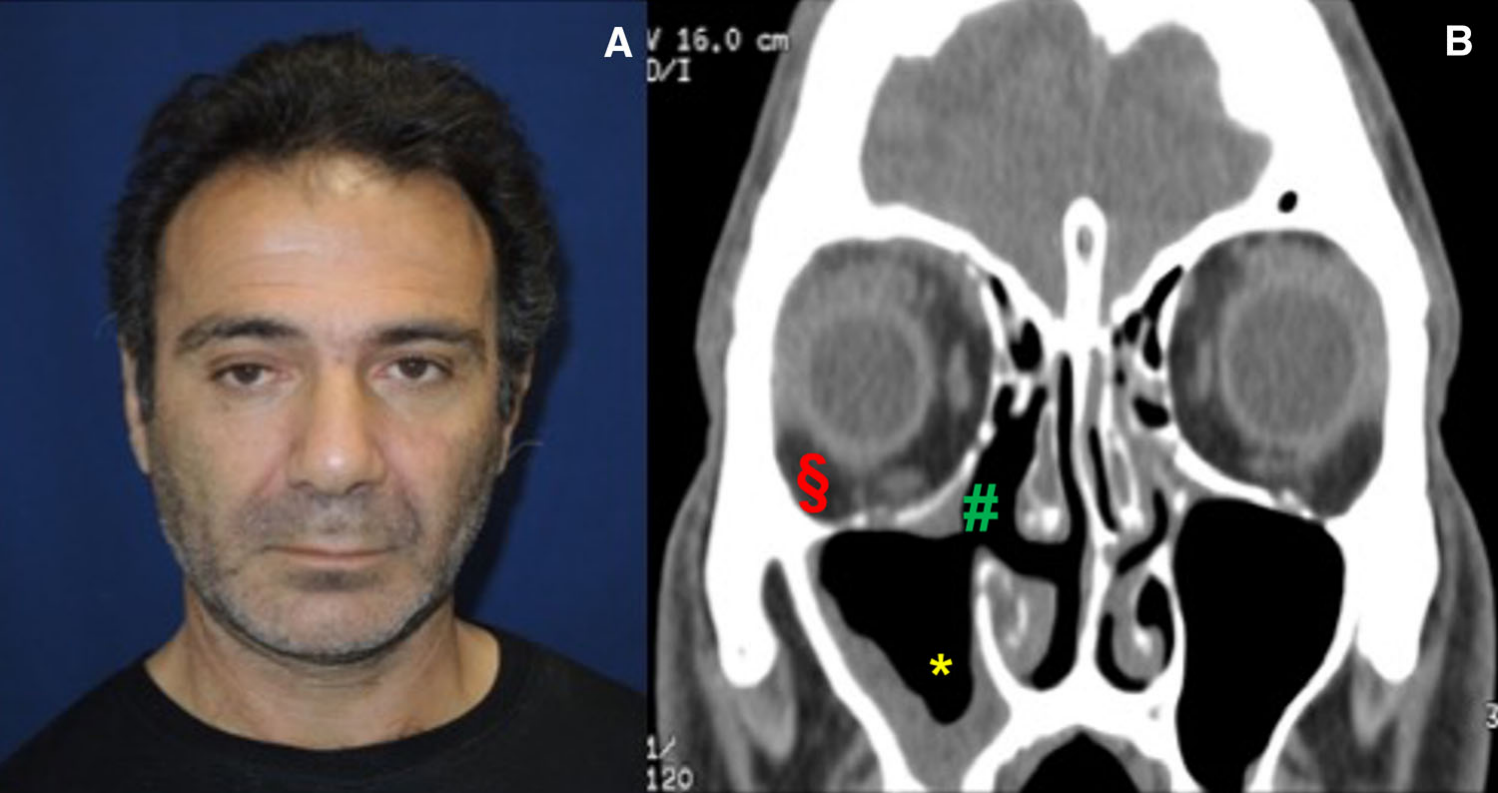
Case 1: Postsurgical photo of patient in frontal section: rehabilitation of consensual extrinsic motility of the eyes (a) and CT Imaging in coronal section: maxillary sinus empty of abscess secretions (*), periorbital edema reduction (§), site of antrostomy (#) (b)
